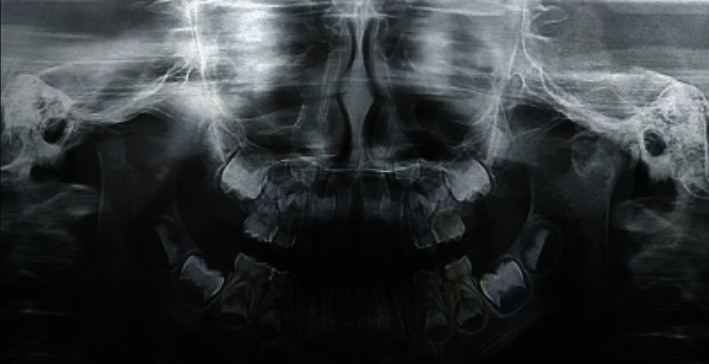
A pre-operative orthopantomography (OPG) of the patient.
